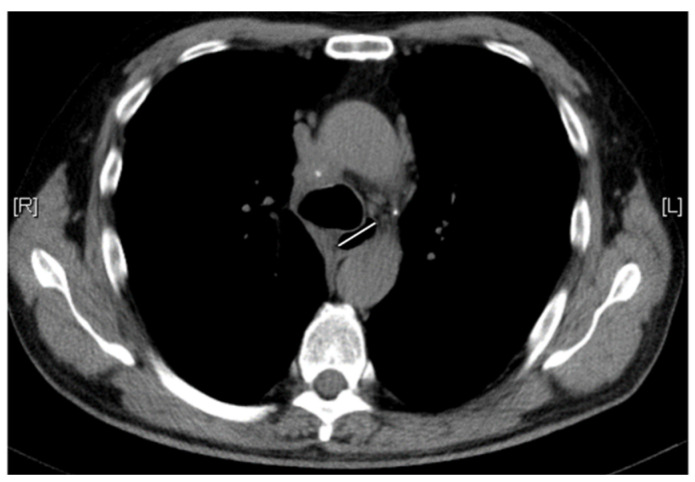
Widest esophageal diameter (WED) assessment (white line) on chest high-resolution computed tomography scans.
